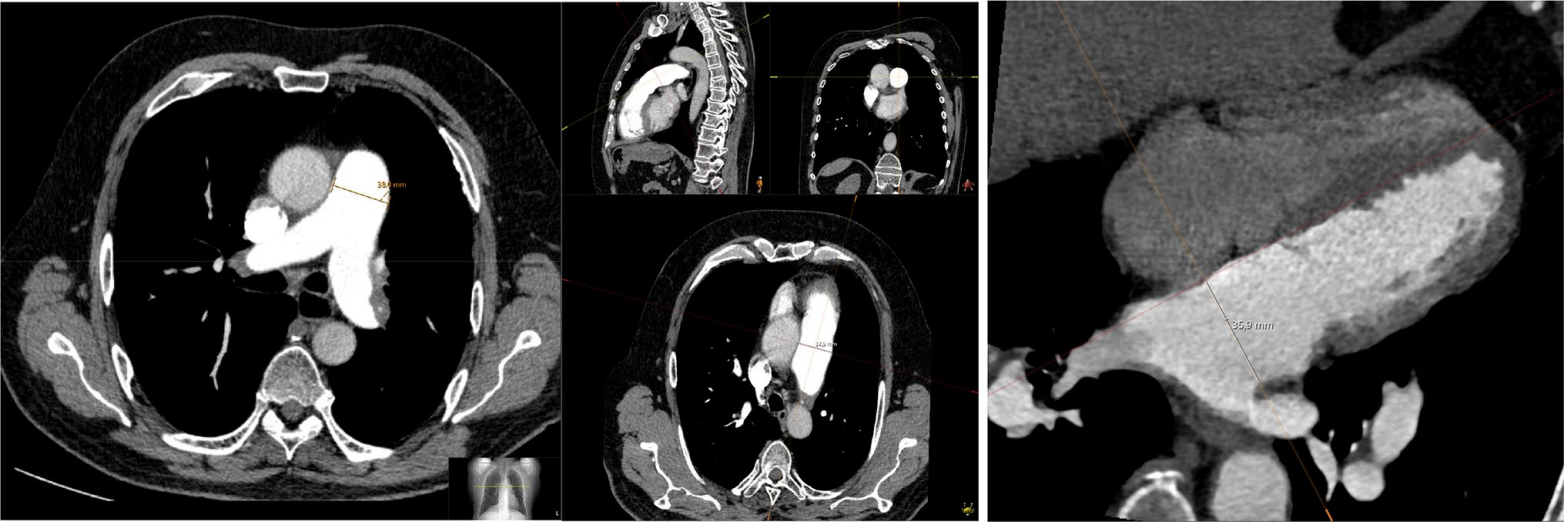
Measurements of computed tomography parameters in axial plane and multiplanar reconstruction. Shown is the different measurement technique in simple axial (left) and multiplanar reconstruction (right) for the same parameter—pulmonary trunc diameter. Difference in pulmonary trunc diameter was 0.1 mm in this patient. Further, the exemplary measurement of the left atrium in MPR is show
